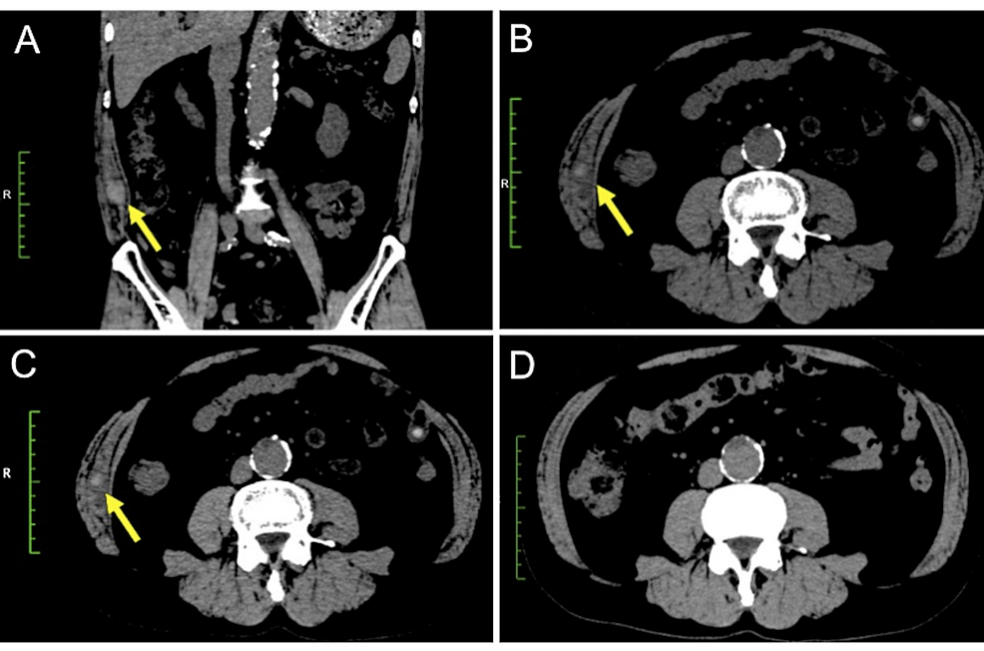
CT findings of the abdomen and pelvis (A, B) CT scan on Day 1 revealed a 22 × 18 × 10 mm right RSH with a high attenuation. RSH does not cross the midline or dissect fascial planes (arrow). (C) CT scan obtained one week after the onset of the illness revealed a reduced RSH size (12 × 11 × 8 mm). Abbreviations: CT, computed tomography; RSH, rectus sheath hematoma
